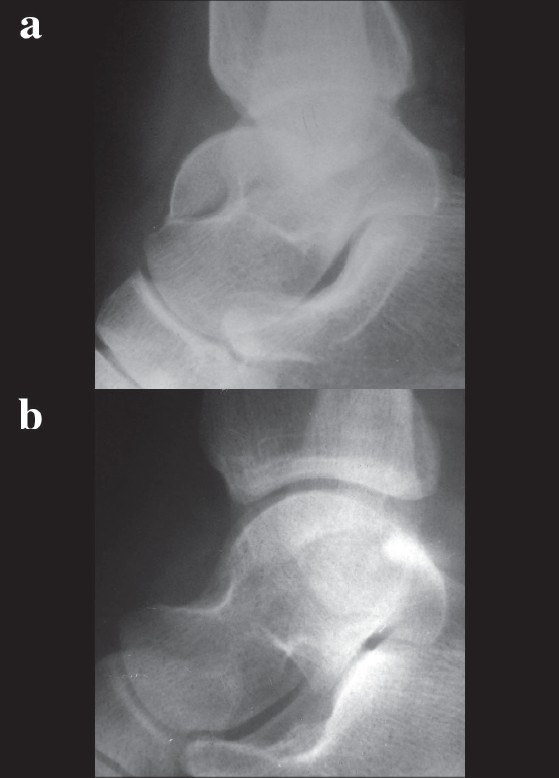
(a) Preoperative X-ray showing fracture talus. (b) 6 months postoperative X-ray shows fracture talus fixed with bio-screws showing excellent reduction and fracture union
